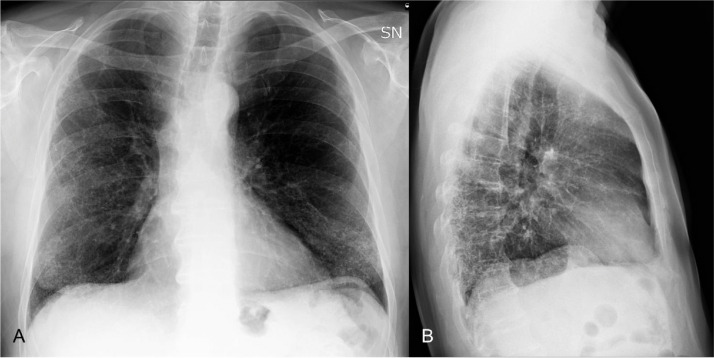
A - B. Chest radiograph, posterior-anterior and lateral view, demonstrating reticulo-nodular pattern involving above all the lower lobes.
